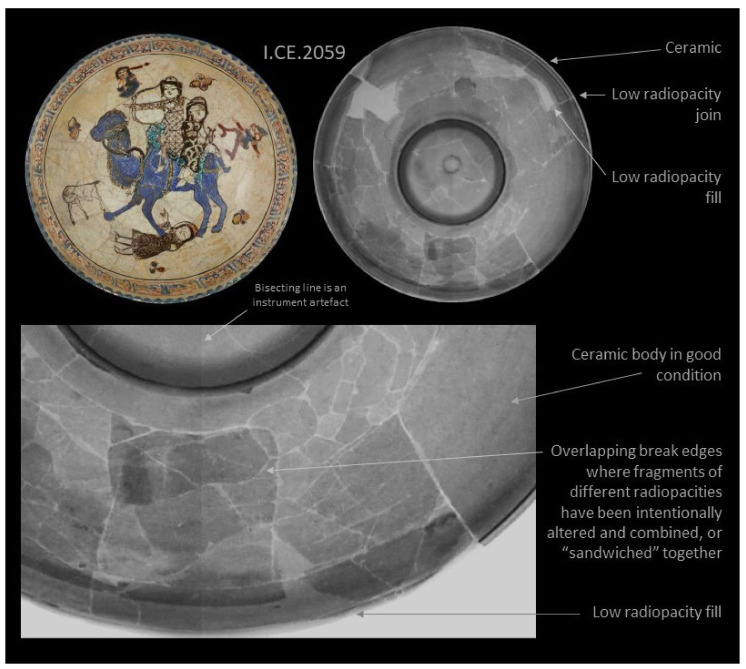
Results for I.CE. 2059, upper left digital photography, upper right digital radiograph, the detail below shows overlapping shards in the recumbent figure’s dress.
